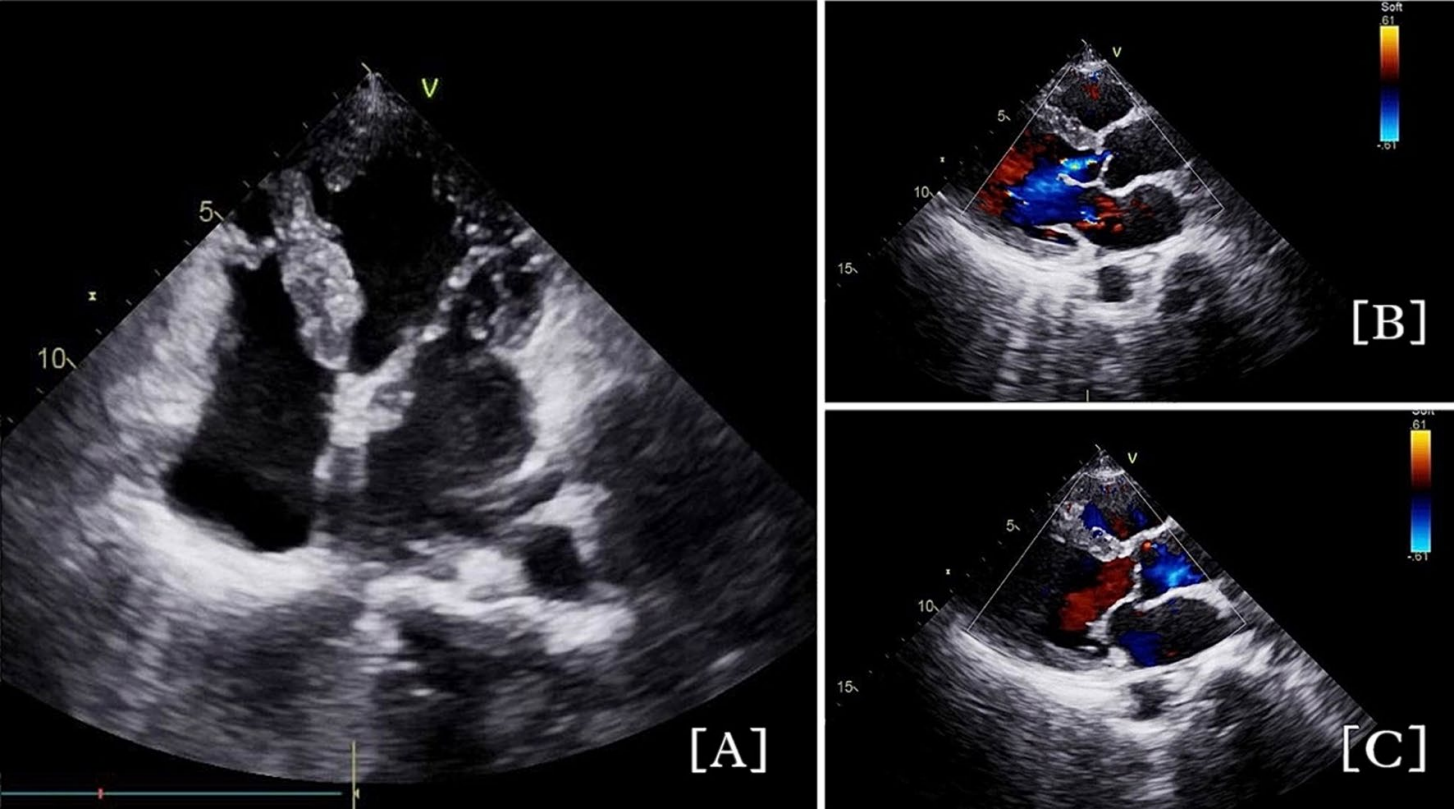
Echocardiogram Images in patients with Takotsubo Cardiomyopathy. A ECHO of a patient after a Bee Sting showing Apical Trabeculation, mild Left Ventricular Systolic Dysfunction, and hypokinetic Left Ventricle. B Doppler ECHO with Systolic Dysfunction. C Doppler ECHO with Normal Diastolic Function
